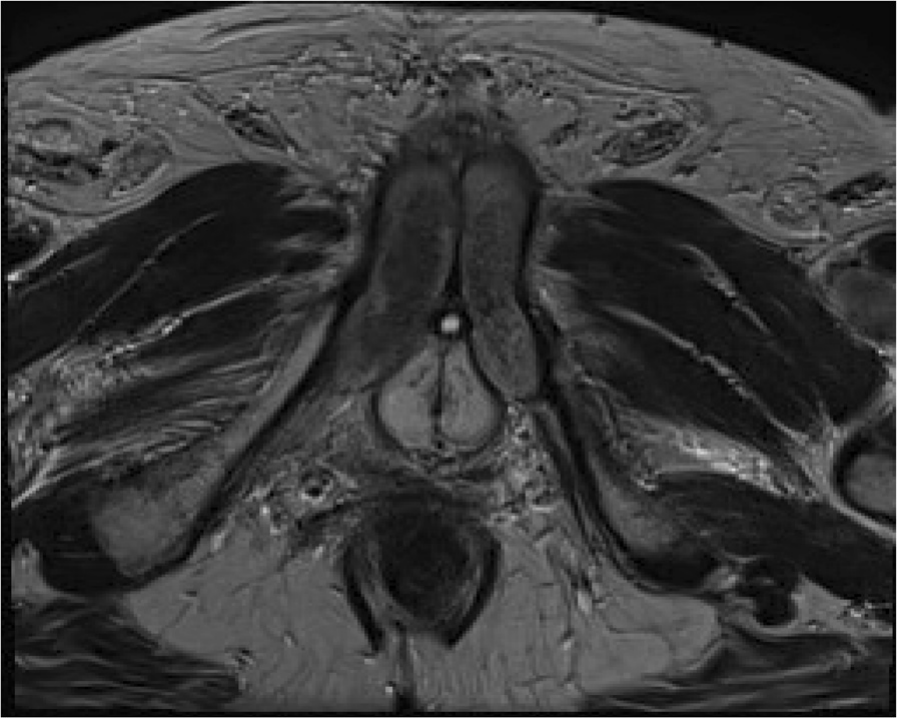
Hyposignal intensity in the right corpus cavernosum on a perineal axial T2-weighted slice
